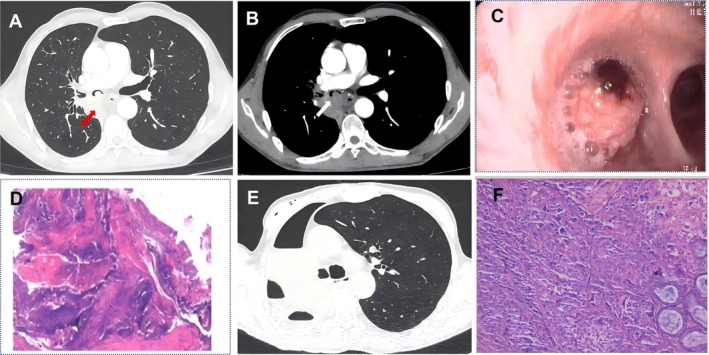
A patient with lung cancer presented with massive hemoptysis and underwent video‐assisted right pneumonectomy with mediastinal lymph node dissection on ECMO support. (A) Contrast‐enhanced CT images revealed right lower lobe mass (indicated by red arrow) and (B) endobronchial mass lesion (indicated by white arrow). (C) Bronchoscopy identified a friable, cauliflower‐like lesion occluding the right middle lobe bronchus. (D) Biopsy confirmed keratinizing squamous cell carcinoma. (E) CT images post video‐assisted right pneumonectomy with mediastinal lymph node dissection. (F) Post‐surgical pathology revealed invasive non‐keratinizing squamous cell carcinoma.
